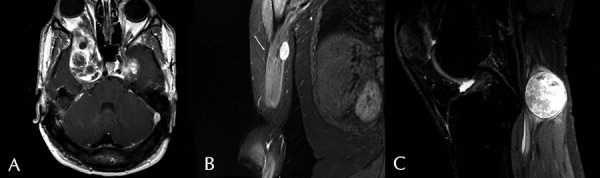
Post-contrast T1 weighted MR image. A: Axial image displaying large enhancing tumors of the cavernous sinus and right orbit (Patient 11). B: Coronal image of right arm showing enhancing tumor (Patient 4). C: Sagittal image of right knee depicting enhancing tumor on right tibial nerve (Patient 3).
